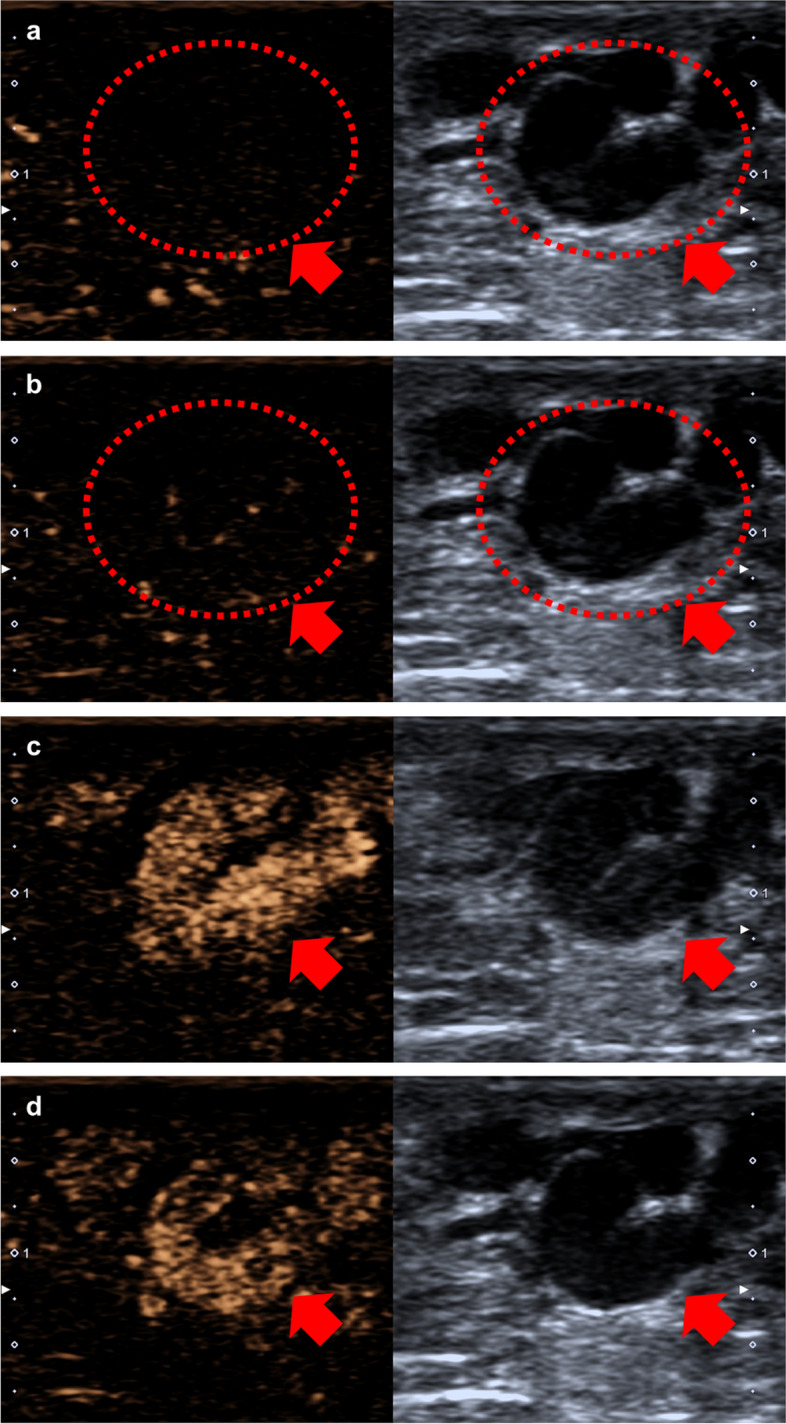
Example of CEUS images. a No microbubbles appeared in the spermatic vein before contrast injection. b Microbubbles began to enter the spermatic vein after contrast injection. The number of microbubbles entering the spermatic vein gradually increased to the peak (c), then gradually decreased (d), and finally became zero. The red circles and red arrows indicate the spermatic vein. CEUS contrast-enhanced ultrasound
